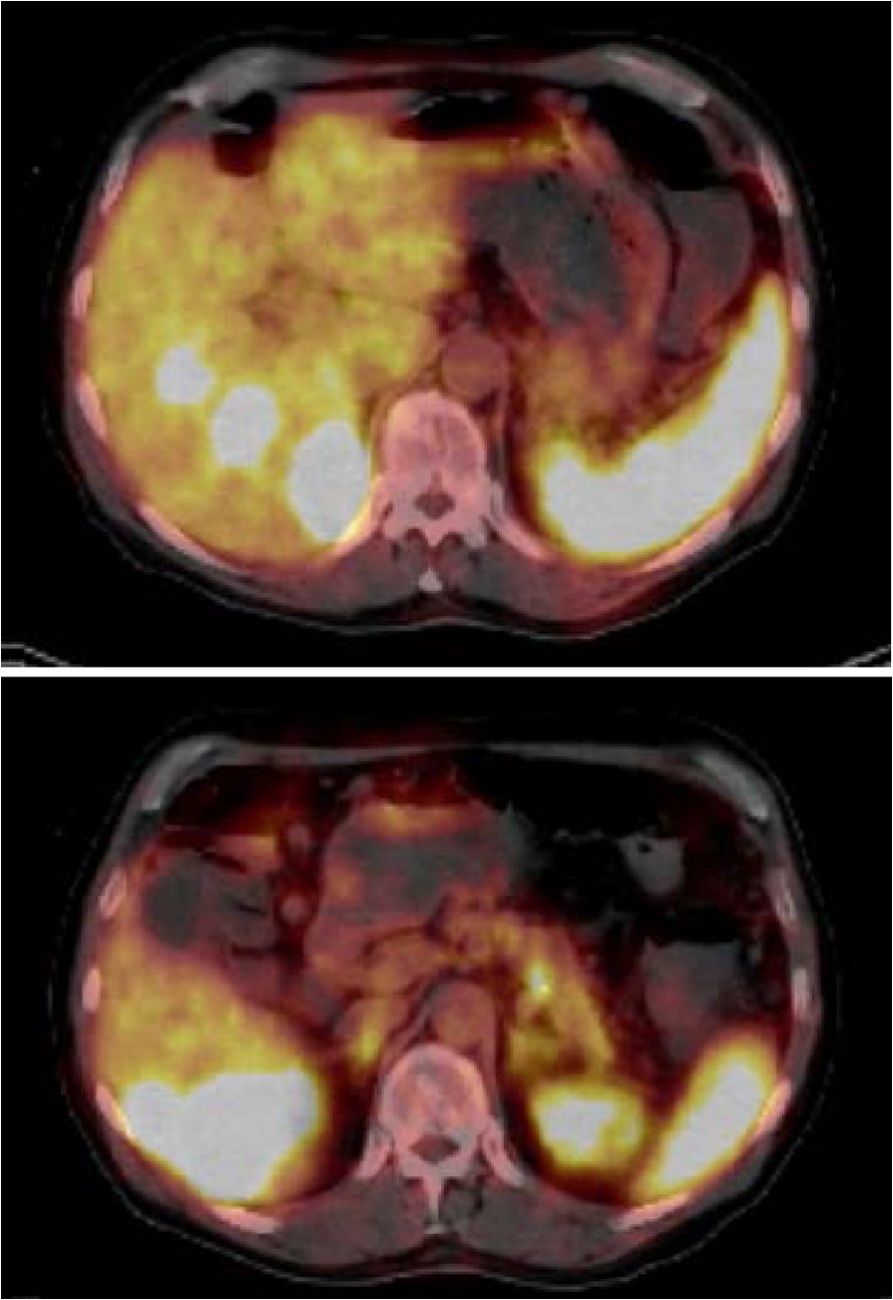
PET-CT with MBq 68-Gallium-DOTATOC showing enhancement of multiple lesions in the right lobe of the liver (upper image) and a slight enhancement in the caudal pancreas (lower image).
